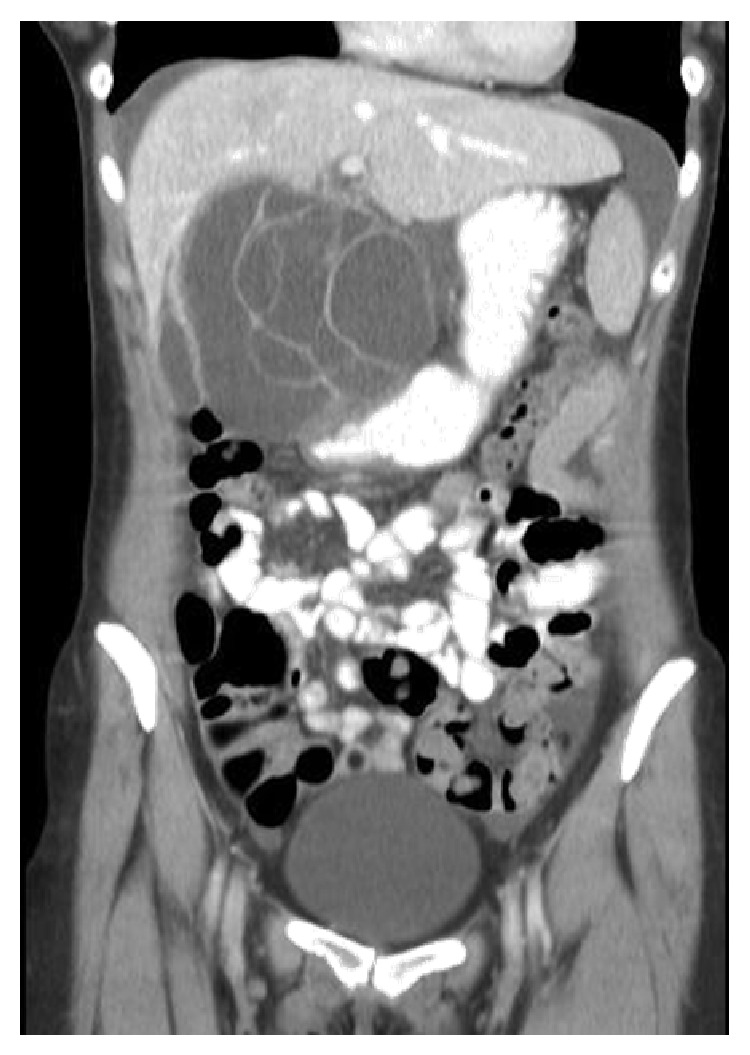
Name of image: CT coronal reformat with intravenous contrast. Description: contrast enhanced CT confirmed the presence of a large complex cystic mass. The origin of the mass was in close proximity to the pancreas, liver, and stomach. No biliary or pancreatic duct dilatation was observed on CT.
